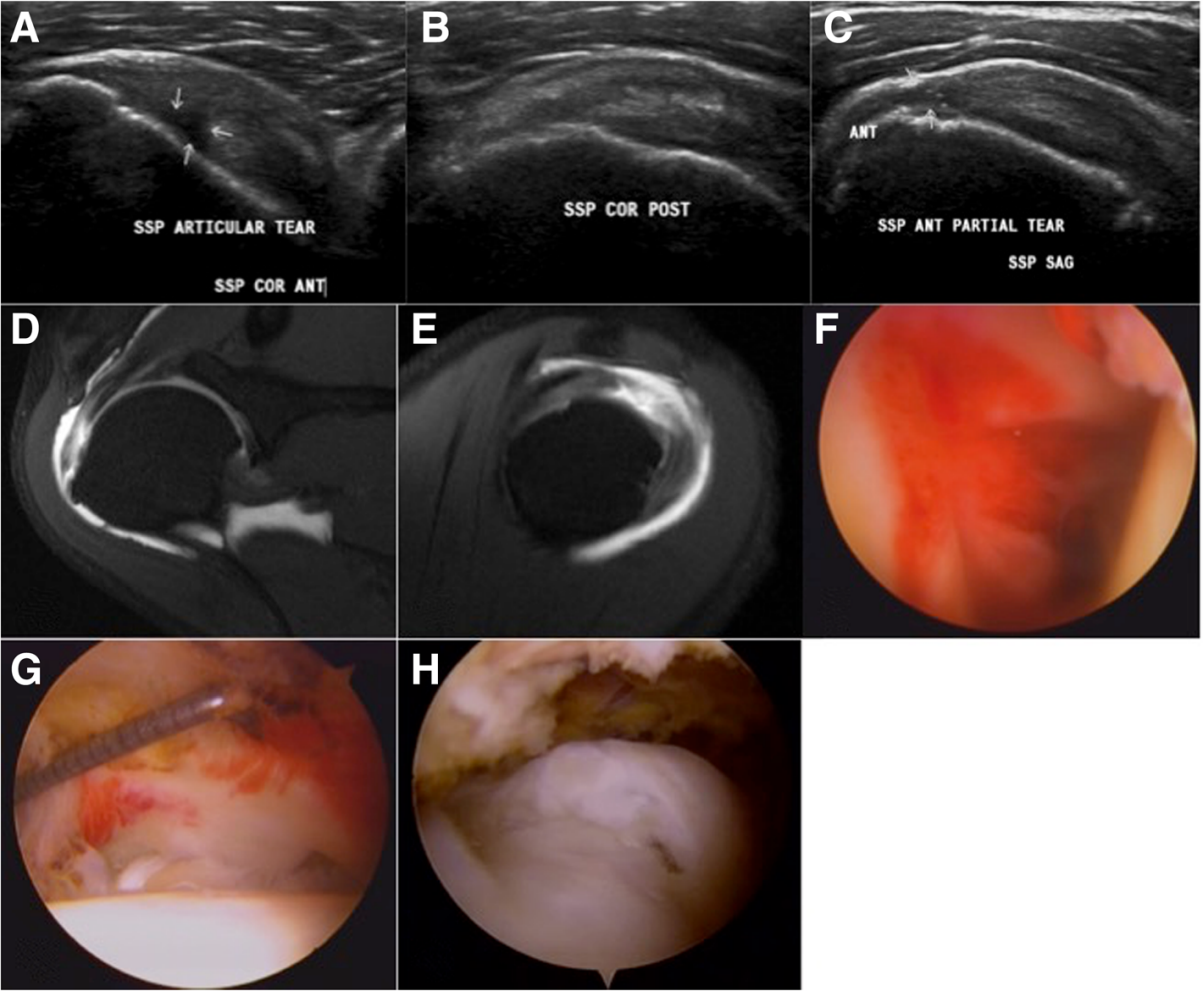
Case 2. A 52-year-old male patient with diabetes had right shoulder stiffness and a near full-thickness rotator cuff tear. a Coronal ultrasonographic view of the anterior part of the supraspinatus. b Coronal ultrasonographic view of the posterior part of the supraspinatus. c Sagittal ultrasonographic view of the lateral part of the supraspinatus. d Coronal view of MRI of the anterior part of the supraspinatus. e Sagittal view of MRI of the lateral part of the supraspinatus. f Intra-articular view of the shoulder adhesive capsulitis. g Intra-articular view of the anterior part of the supraspinatus tendon near the full-thickness tear. h Subacromial view of the anterior part of the supraspinatus tendon near the full-thickness tear
